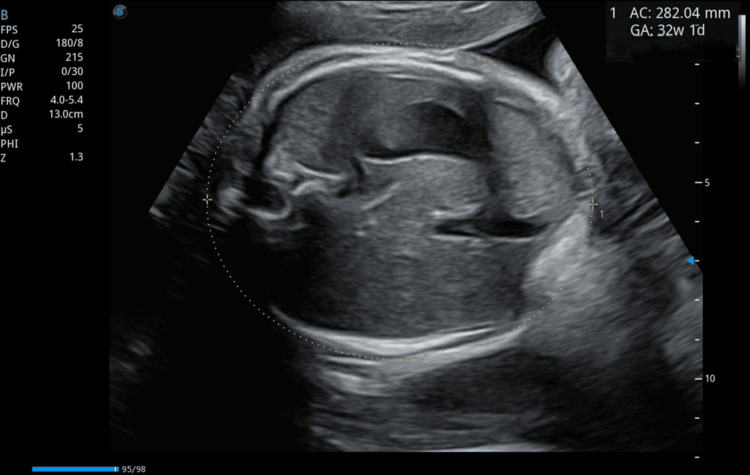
Ultrasound image demonstrating a transverse section of the fetal abdomen at 32 weeks and one day of gestation
